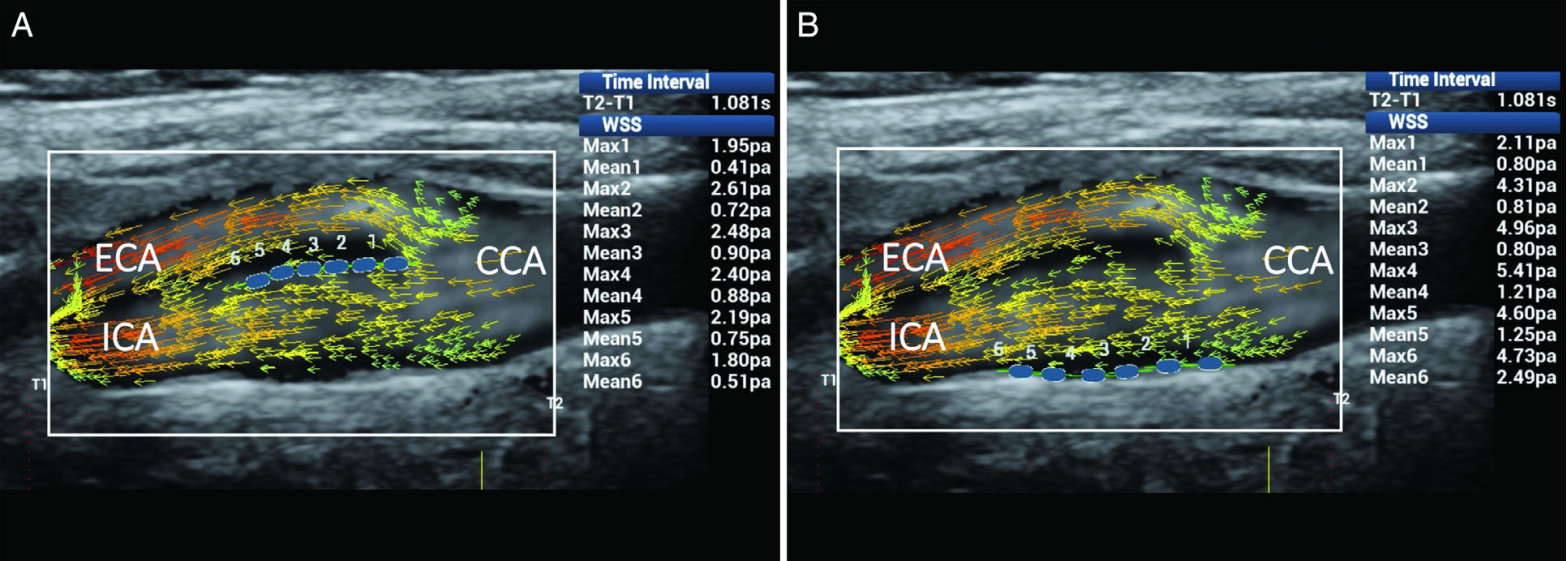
Mainly laminar flow in the CB. Ultrasound vector flow imaging shows the vector direction remaining parallel to the vessel walls. Wall shear stress measurements (blue dots) gave maximum and mean values within the normal range: in particular, along the flow divider, 1.80 to 2.61 and 0.41 to 0.90 Pa, respectively (A), and along the opposite wall from the flow divider, 2.11 to 5.41 and 0.80 to 2.49 Pa (B). CCA indicates common carotid artery; ECA, external carotid artery; and ICA, internal carotid artery.
